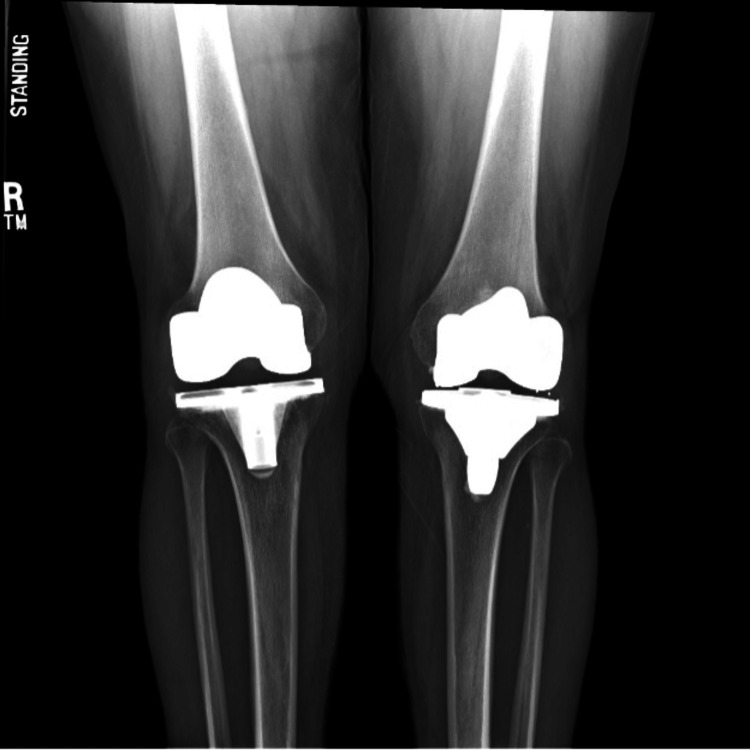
Bilateral AP X-ray of the knees.
